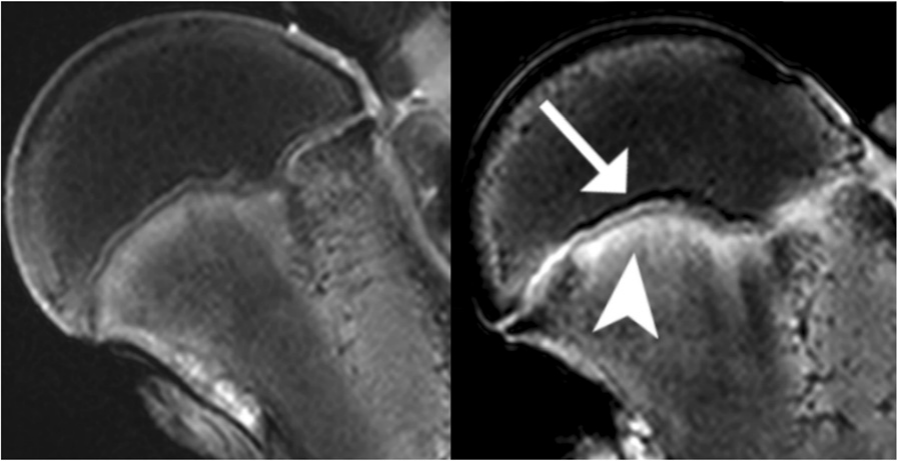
MRI image of the control hip (left) and a vertically loaded hip (right). After loading, difference in signal intensity was seen medially to the epiphyseal tubercle. A lower signal was seen above the epiphyseal line (white arrow) and higher signal below the epiphyseal line (white arrowhead).
